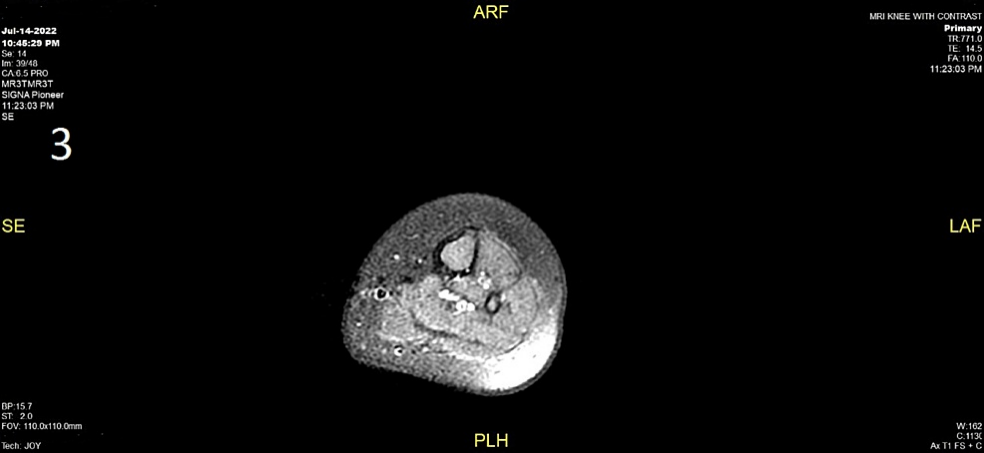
MRI of the left knee joint This figure demonstrates the synovial thickening and enhancement with mild knee joint effusion and surrounding soft tissue edema. The visualized bones show no significant bone marrow edema, periosteal new bone formation, or abnormal enhancement.
